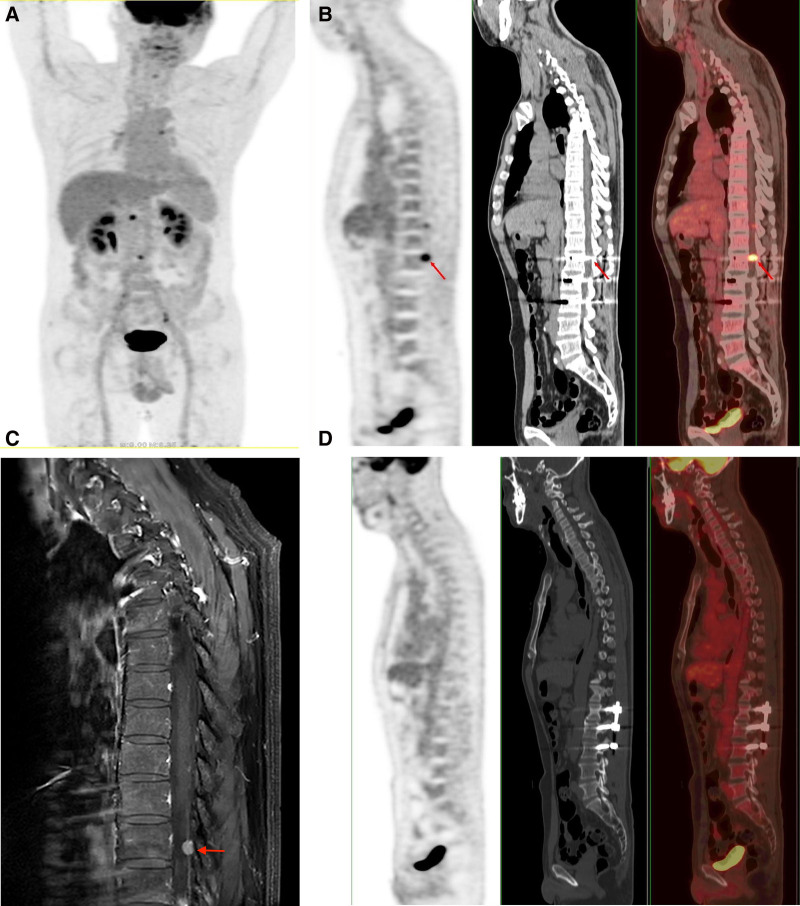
PET-CT scan images and MRI. (A) Fist postoperative PET-CT scan showing no primary lesions in other parts of the body. (B) Fist postoperative PET-CT scan showing a metastatic nodule in the spinal canal. Red arrow = metastatic nodule. (C) Fist postoperative MRI showing a metastatic nodule in the spinal canal. Red arrow = metastatic nodule. (D) Second postoperative PET-CT scan confirming total surgical resection of the spinal lesion and spinal fixations. CT = computed tomography, MRI = magnetic resonance imaging, PET = positron emission tomography.
